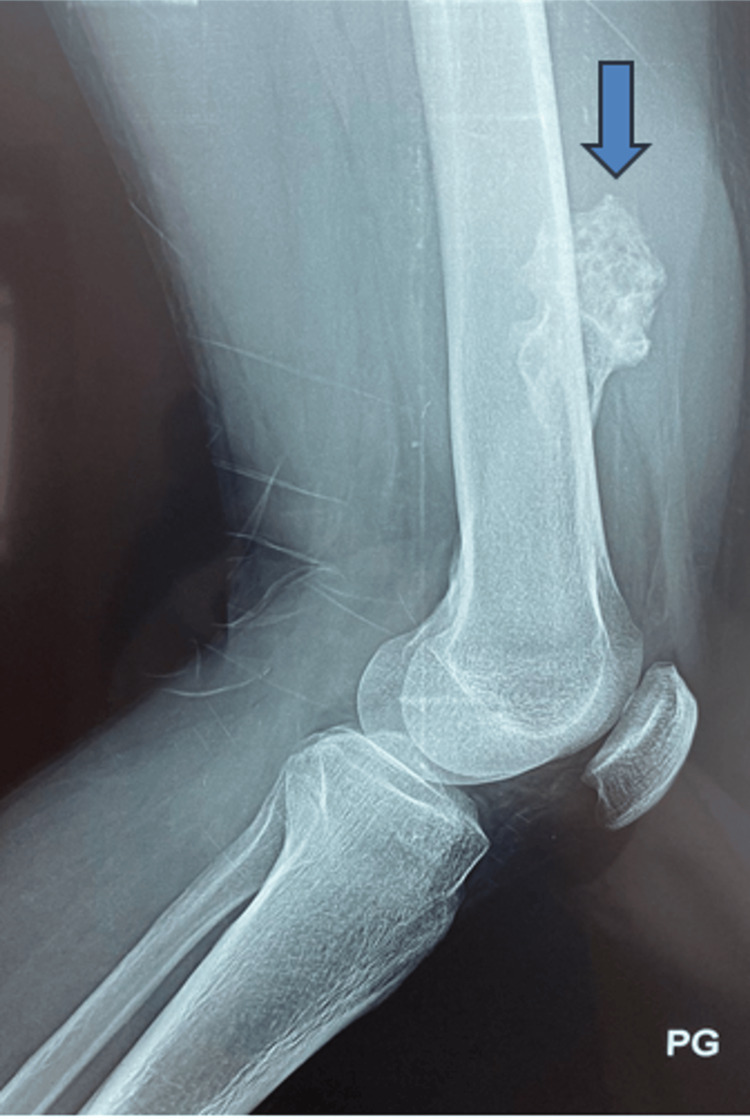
Standard radiograph before osteochondroma resection.
